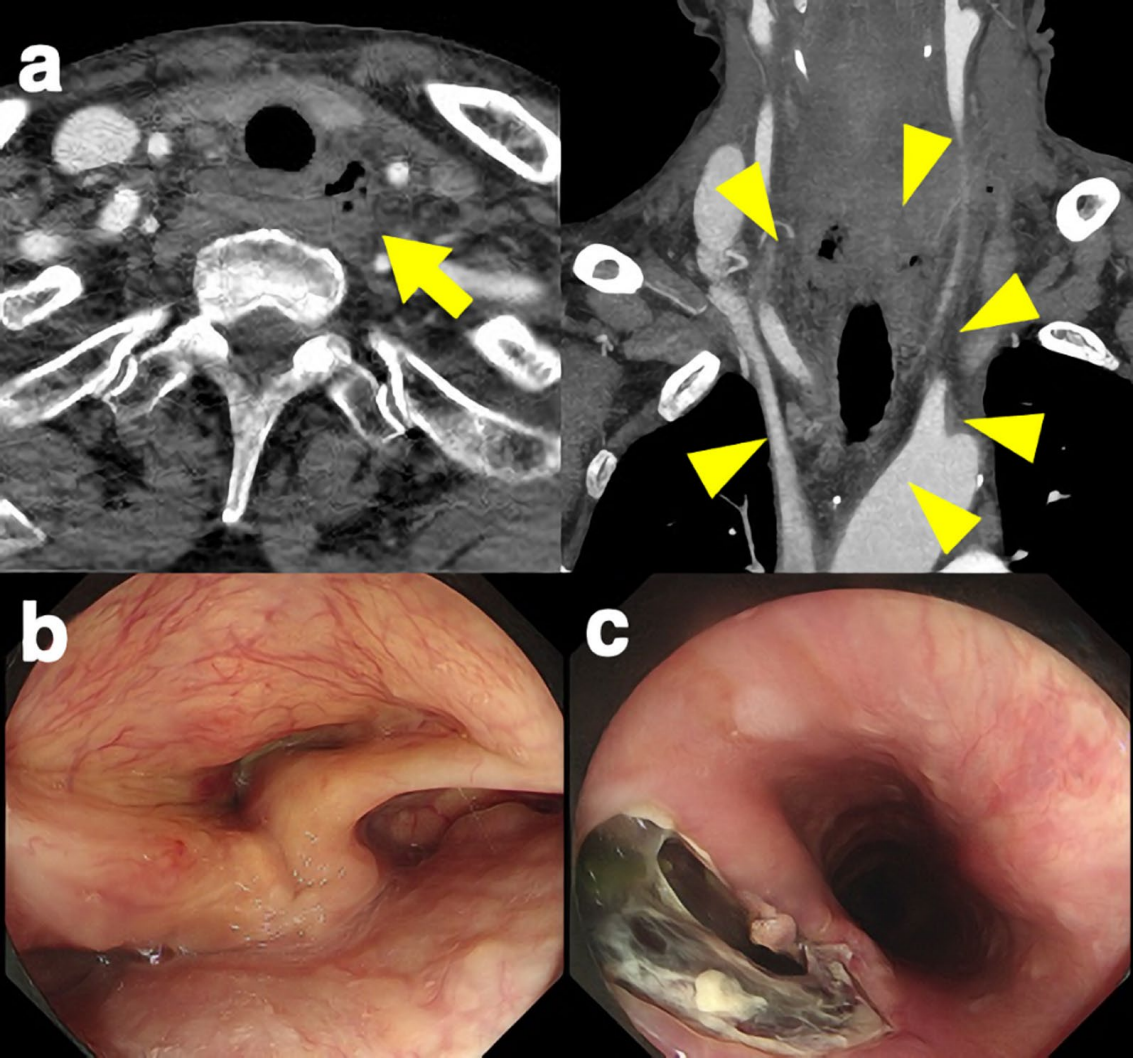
Examinations on the second day after the injury. CT revealed free gas (yellow arrow) and fluid accumulation (yellow arrowheads) around the thoracic esophagus (a). Upper gastrointestinal endoscopy shows left vocal cord palsy (b) and injury site on the esophageal wall (c)
